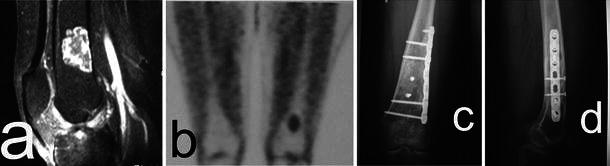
a Grade 1 CS of the distal femur. MRI lateral view. b Positive positron emission tomography (PET) scan. c, d AP and lateral (LL) radiographs at 5.5 years from surgery. Curettage, filling with allografts, and plate and screws fixation were performed
